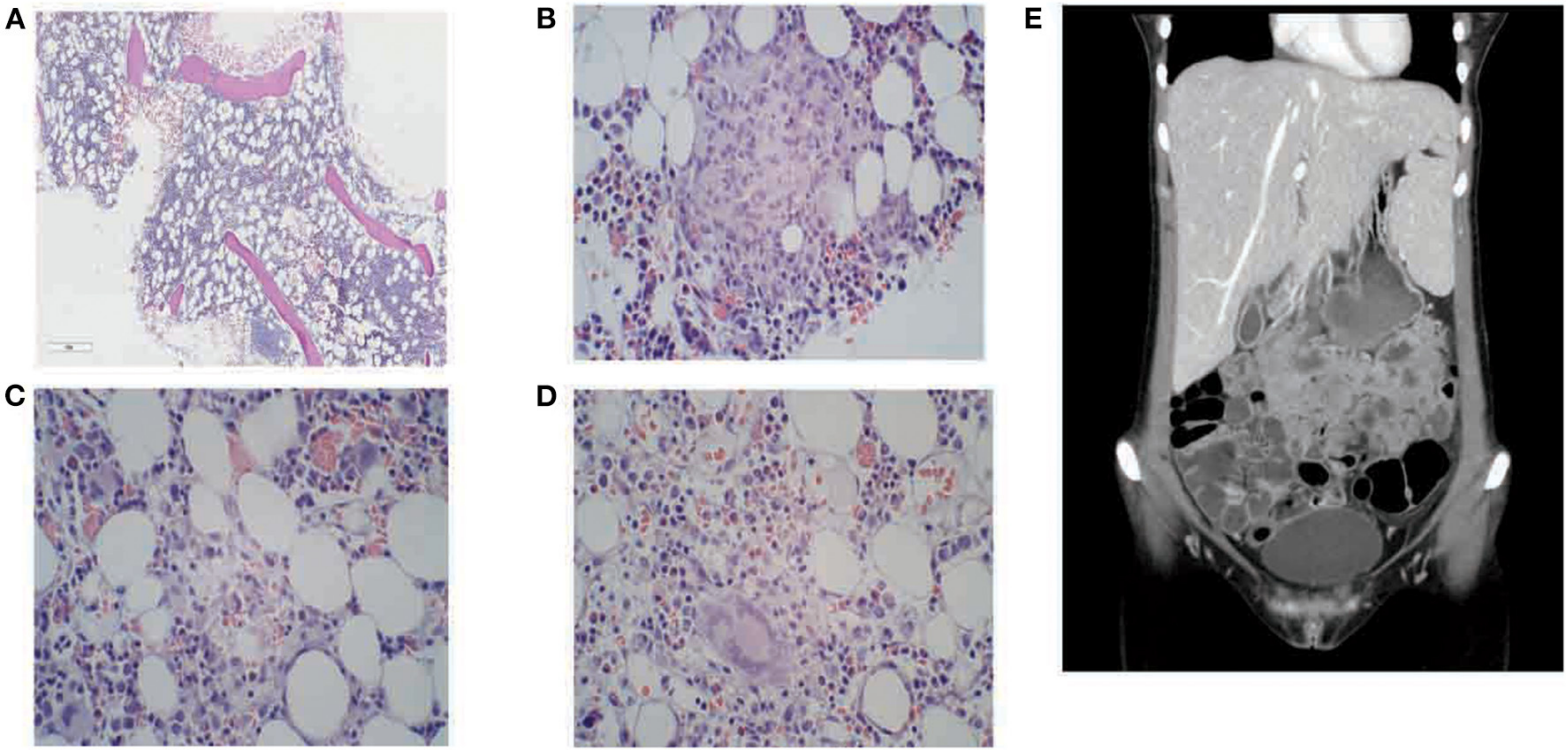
Bone marrow biopsy and CT examination. Bone marrow biopsy from the patient demonstrating; (A) modest hypocellularity; (B) higher magnification demonstrating granuloma formation with inflammation; (C) macrophages with marked hemophagocytosis; (D) giant cells. (E) CT abdomen showing hepatosplenomegaly with “kissing sign”.
